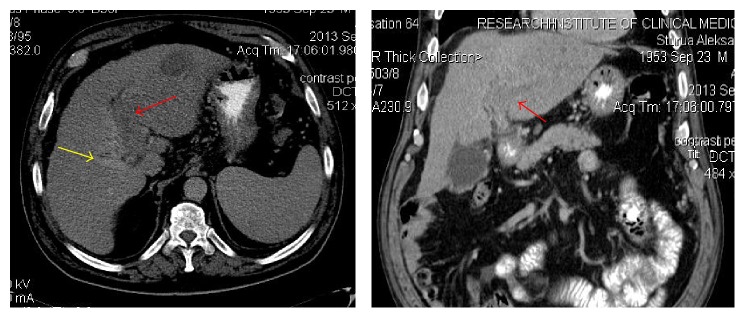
Preprocedure CT (portal phase): RPV patent branch (puncture “target” on VesOpen procedure), yellow arrow; completely obliterated LPV, red arrow.
